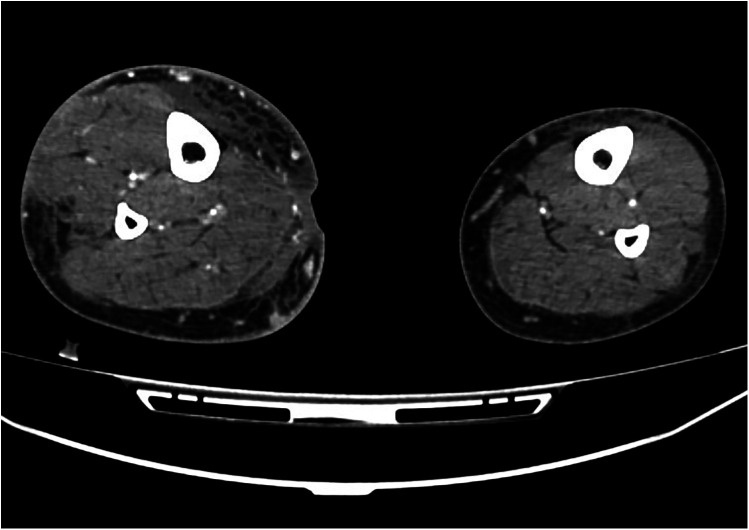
Computed tomography angiography revealed a micro arteriovenous fistula (AVF) in the right leg.
